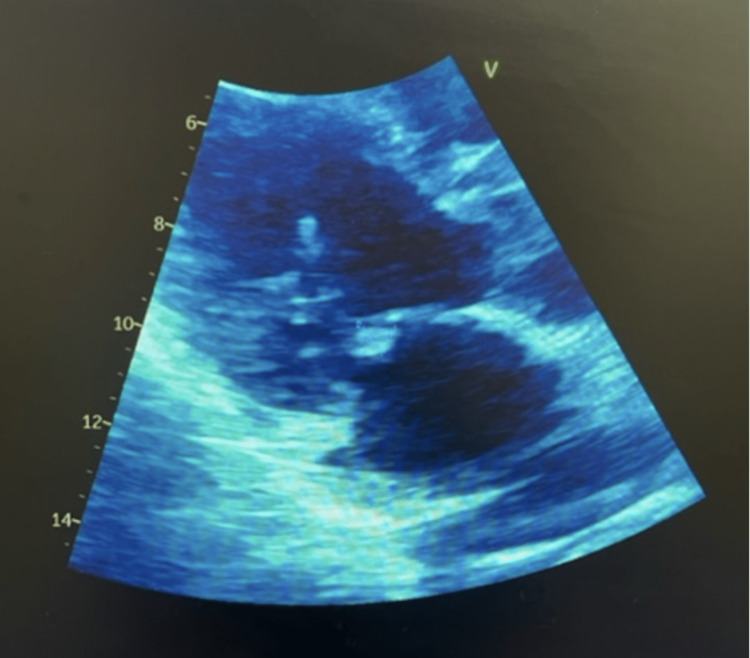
Still from transesophageal echocardiography demonstrating mitral valve vegetations.
